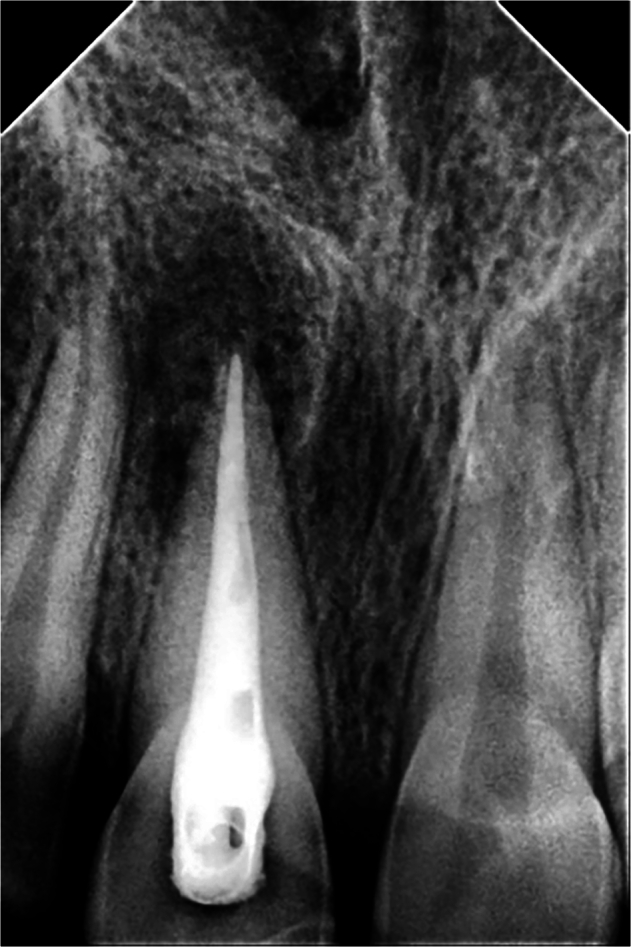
Post-operative periapical radiograph to ensure the obturation quality.
